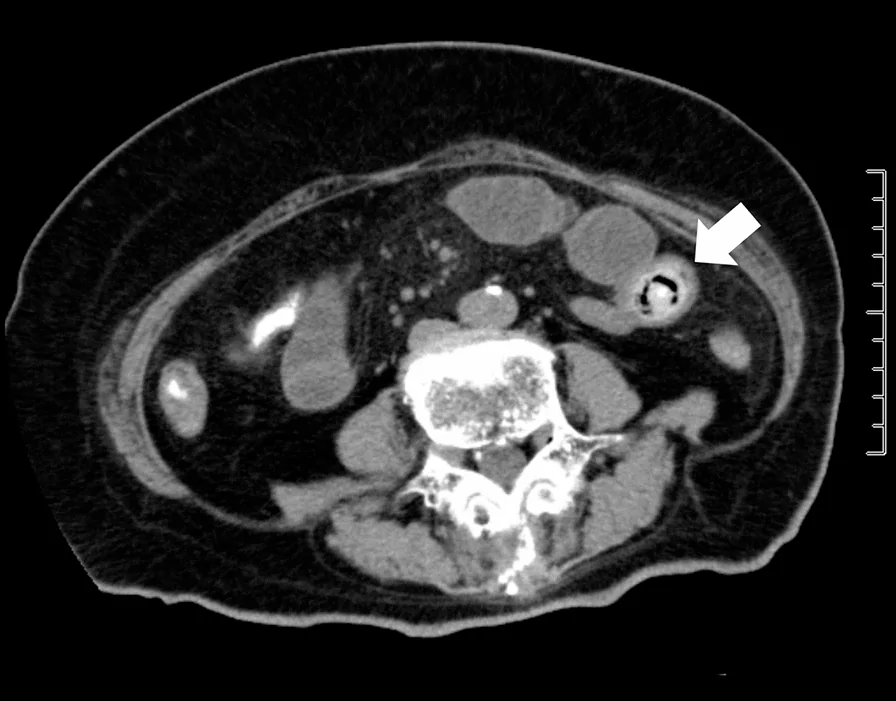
Abdominal CT scan two days after the second ERCP revealing ileus with caliber change at foreign body in the ileum (arrow)
